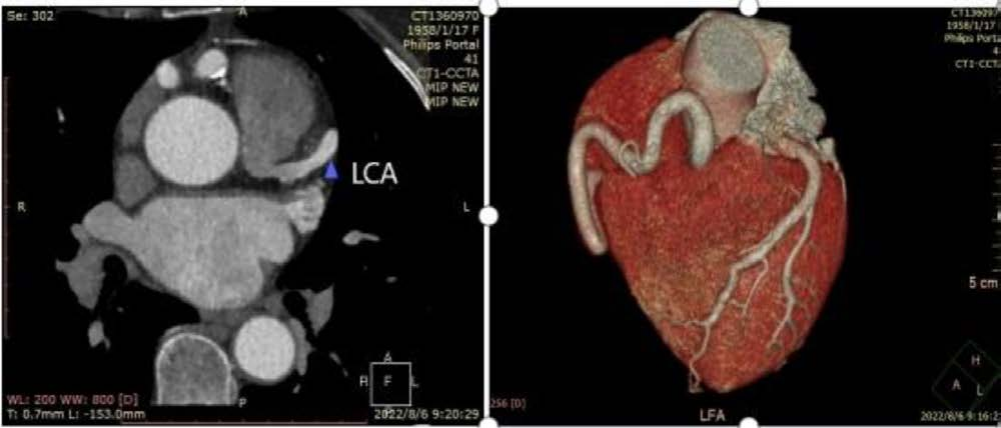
MDCT: The RCA is tortuous and thick, which originates from the aorta and The LCA originates from the pulmonary artery abnormally. LCA = left coronary artery, MDCT = multi-detector computed tomography, RCA = right coronary artery.
